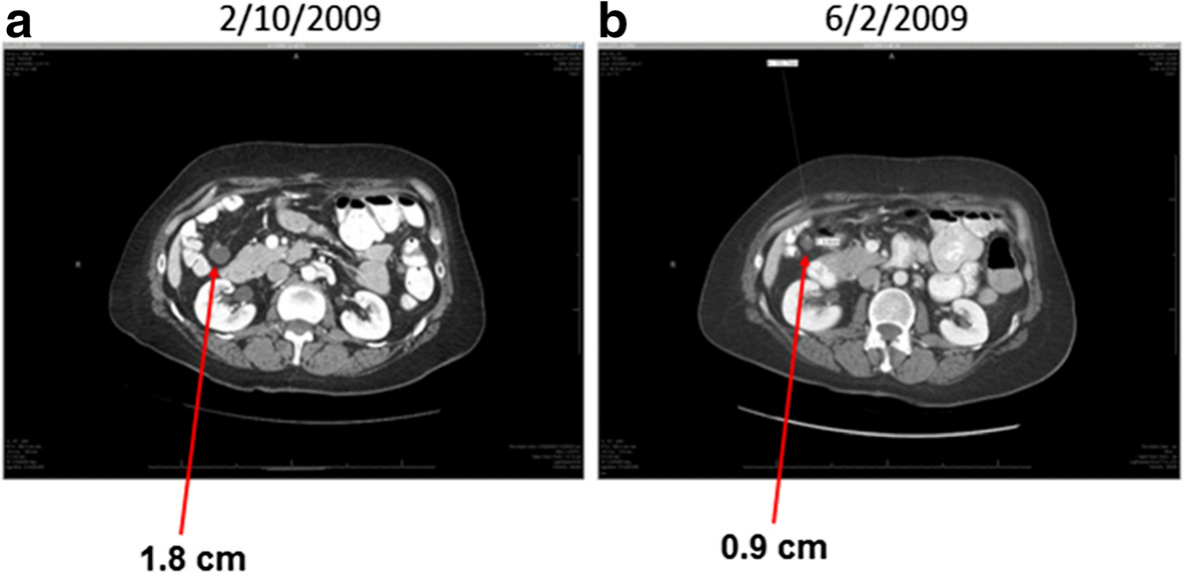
Computerized tomography (CT) scans showing a decrease in an implant close to the hepatic flexure of the colon after the patients have started taking selumetinib since June 2008
